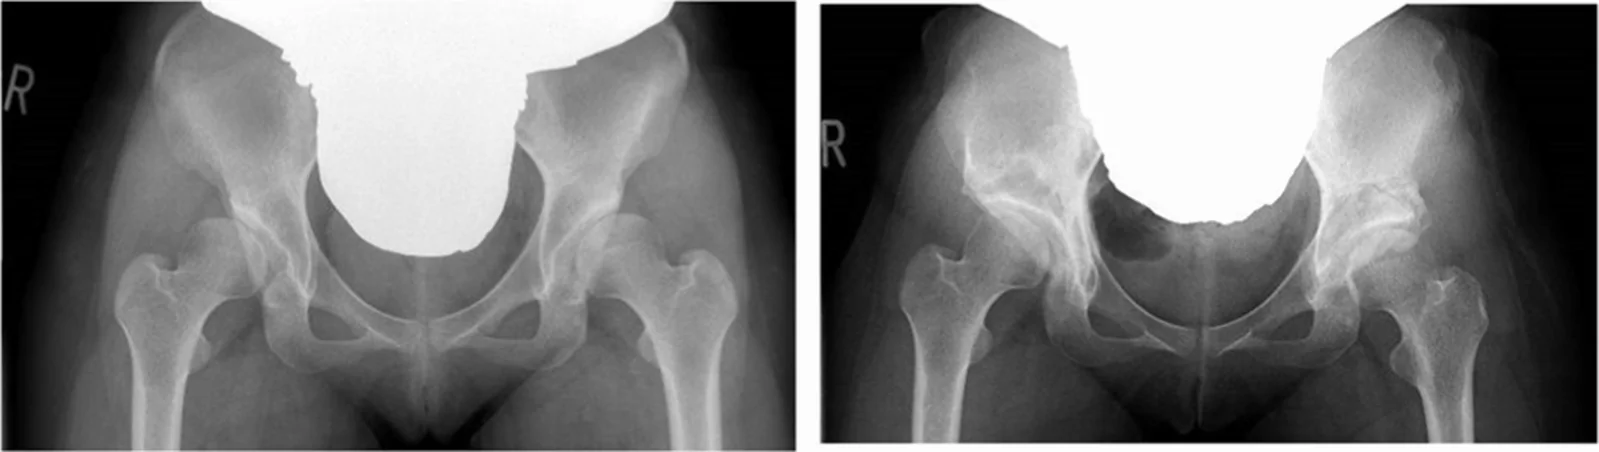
Preoperative and postoperative radiographs of a representative bilateral RAO case. The postoperative radiograph of the right hip was obtained 3 years after surgery, demonstrating complete union of the osteotomy site. The postoperative radiograph of the left hip was obtained 3 months after surgery, when the osteotomy line remains visible before complete union. Modified from Tanaka T et al. [45] under the terms of the Creative Commons Attribution 4.0 International License (CC BY 4.0)
